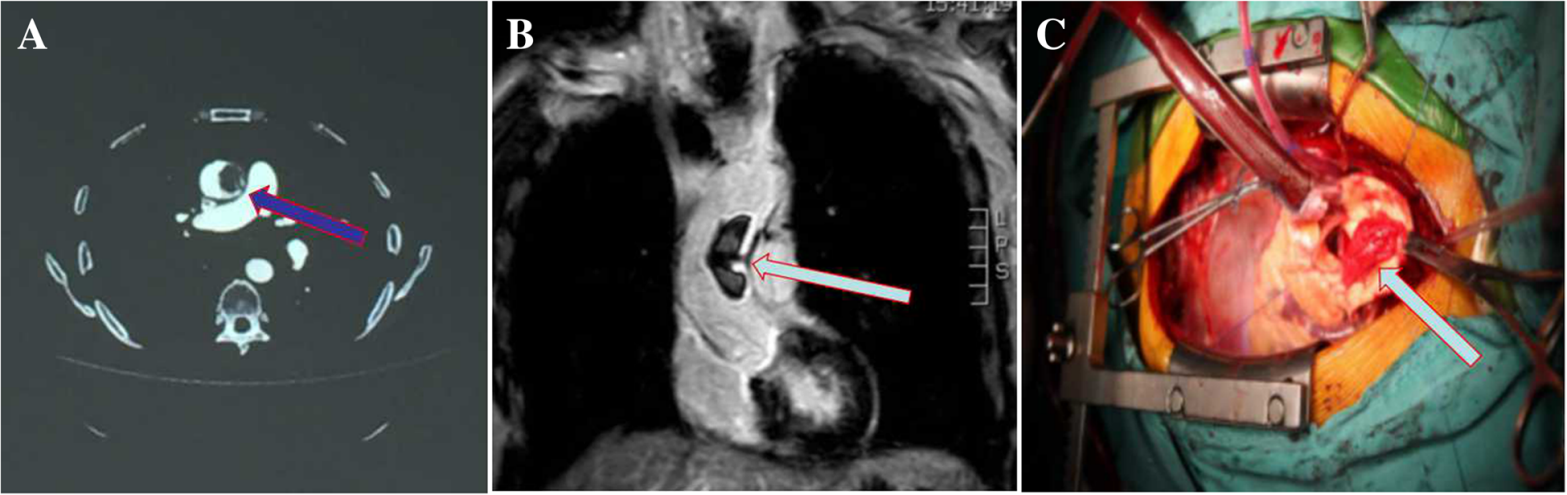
a: Computed tomography showed a large lesion atteched to the aortic inner wall. b: Magnetic resonance investigation showed that the feature of the lesion might be thrombus. c: Intraoperative photography after excision showed the in situ mass attached to the aortic wall (the blue arrow)
